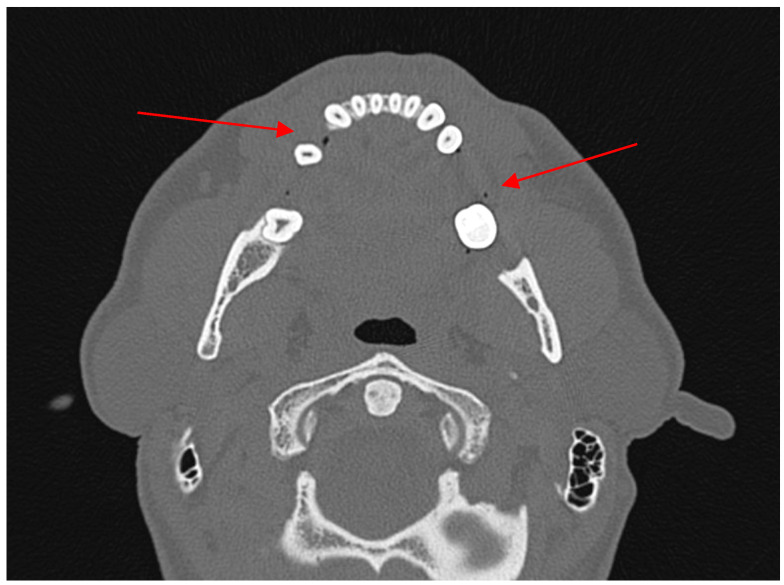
A 47-year-old patient diagnosed with a fracture of the right condylar process of the mandible. The CT scan reveals the presence of air bubbles at the level of the necks and crowns of the teeth (red arrows). Given their position, it can be determined that these are air bubbles associated with saliva in the oral cavity.
